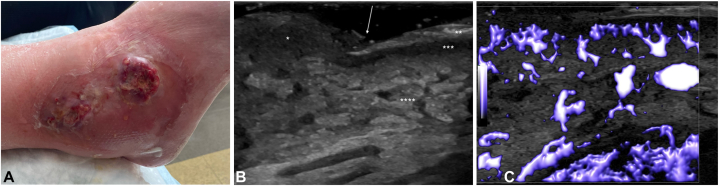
A, Recurrent PG ulceration on right external ankle. B, HFUS with hypoechoic wound (∗) bulging up through skin; hyperechoic epidermis (∗∗) and hypoechoic dermis (∗∗∗) with abrupt disruption of tissue layers at the ulcer edge (*arrow*); cobblestoning (interstitial edema) in the subcutaneous layer (∗∗∗∗). C, HFUS microvascular imaging showing highly vascular wound bed with acute vessel angle branching. *HFUS*, High frequency ultrasound; *PG*, pyoderma gangrenosum.
